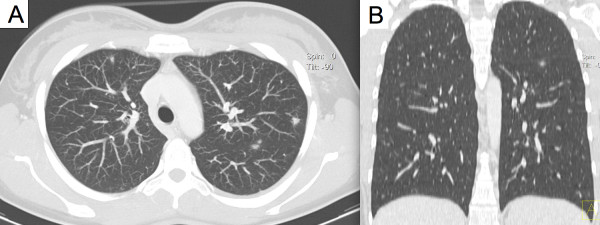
Radiographic findings of lung computed tomography during Katayama syndrome (axial (A) and coronal (B) reconstruction).
